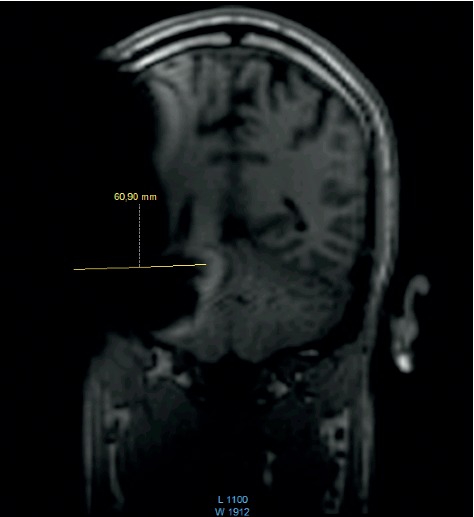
MRI survey pattern of the coronal plain of a 1.5 T scanning of a Medel Synchrony Implant.
